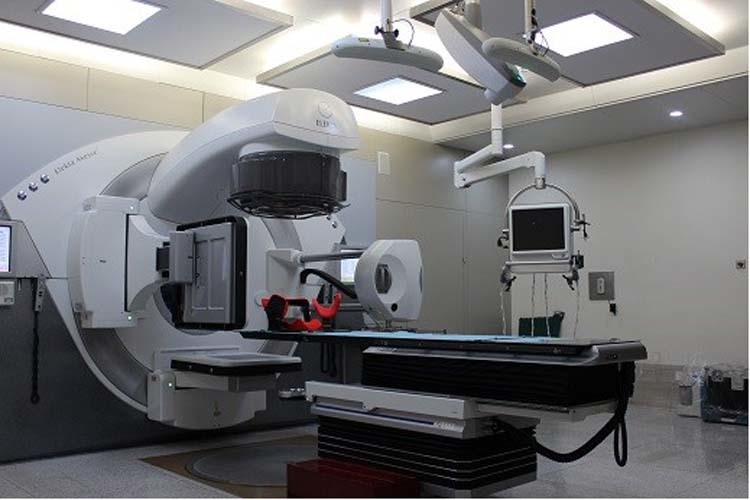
The image of Sentinel and CBCT positioning correction equipment.
